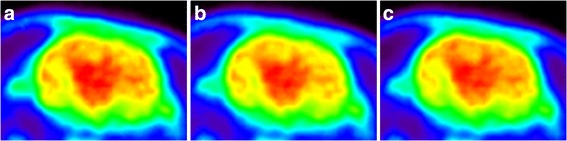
^18^F-FDG PET reconstructed using three different attenuation corrections: *CT-AC* (a), *NoBone-AC* (b), and ^*31*^
